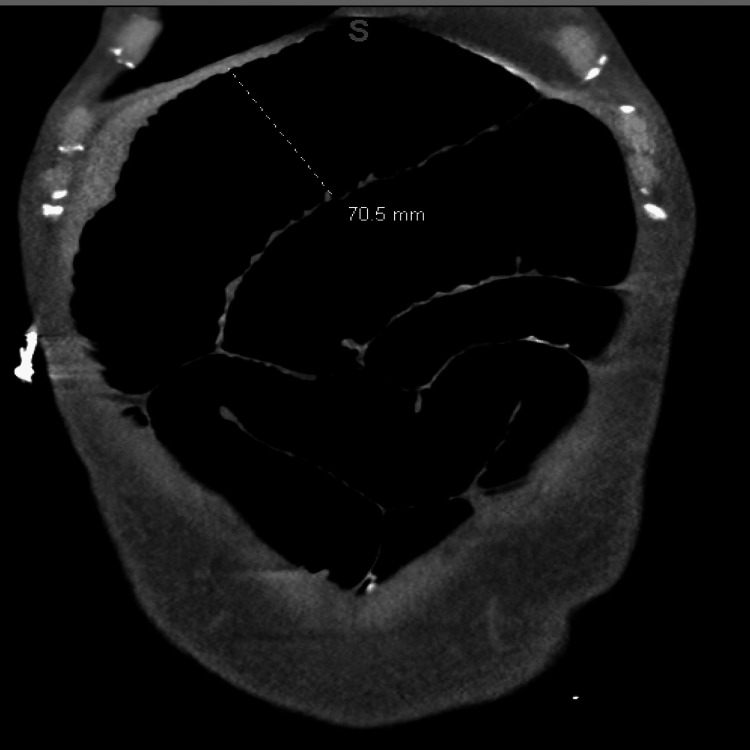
CT scan of the abdomen and pelvis with contrast showing dilated small bowel with no transition point up to 7 cm.
